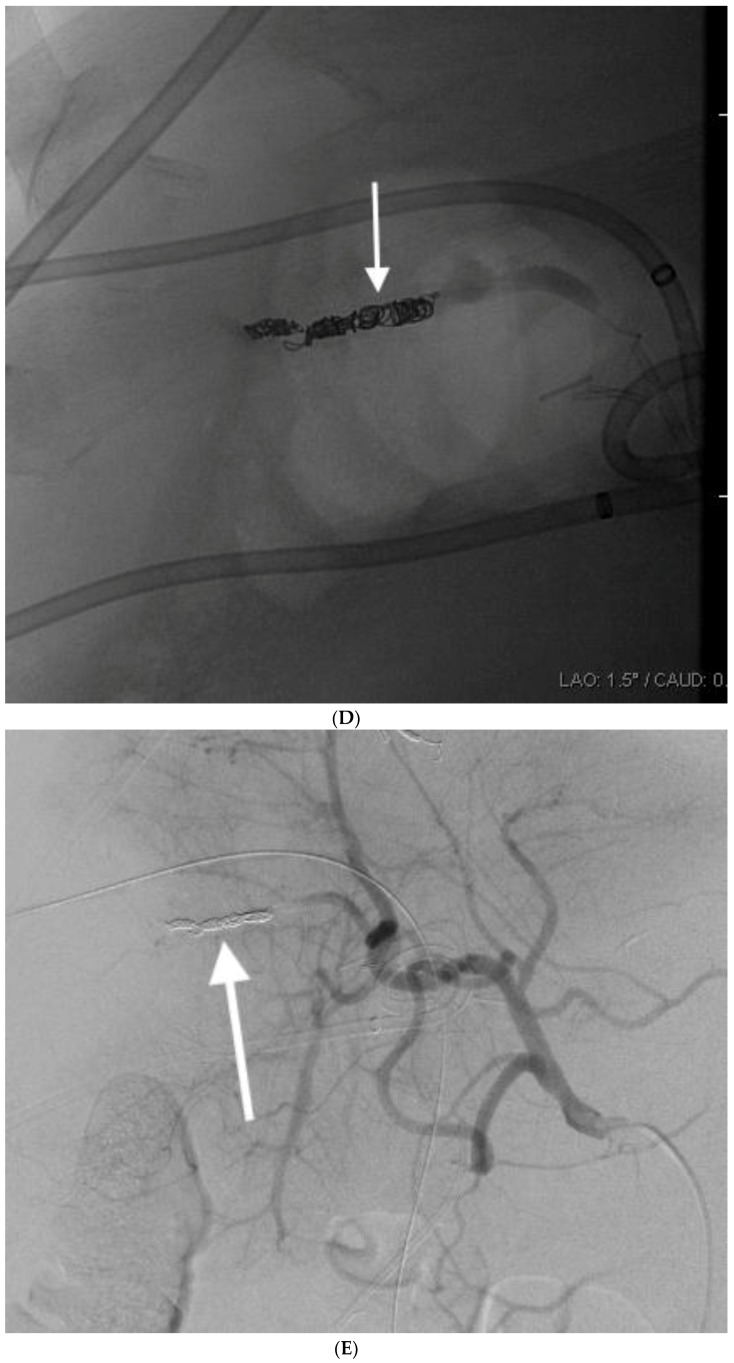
72-year-old male with a hepatic artery to portal vein fistula. This patient underwent PTBD placement for biliary diversion after a cholecystectomy led to a cystic duct stump leak. He had intermittent episodes of small volume hemobilia. (A) Computed tomography angiography (CTA) was performed and showed early opacification of a portal vein branch (curved arrow) for which he was referred for hepatic arteriogram. Celiac (B) and selective right hepatic (C) artery angiograms demonstrated hepatic artery (straight black arrows) to portal vein (curved black arrows) fistula, relatively remote from the PTBD. This injury was therefore thought to be related to initial needle passes. (D) The hepatic artery supplying this fistula was coil-embolized (white arrows). (E) Repeat hepatic angiogram demonstrated cessation of the arteriovenous fistula.
